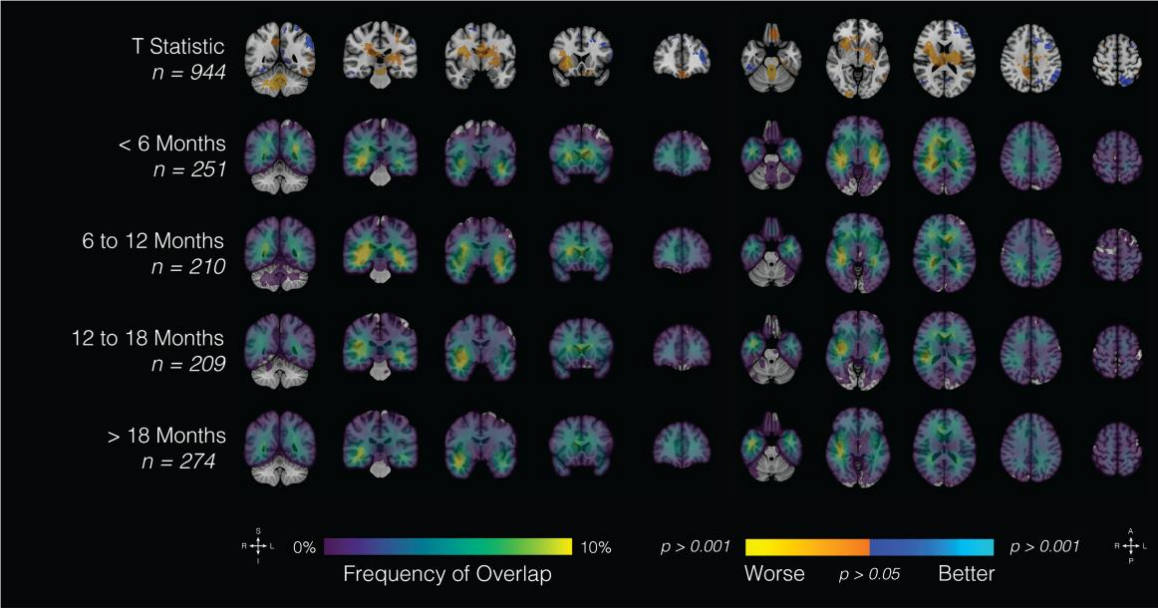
Overall survival and location. Probabilistic maps showing spatial distribution of gliomas by survival. Results are overlaid on the coronal (left half of the image) and axial (right half of the image) T1-weighted MNI brain. Scales indicate *t*-values (first row) and frequency of tumour overlap in percentages (bottom four rows). MNI, Montreal Neurological Institute.
